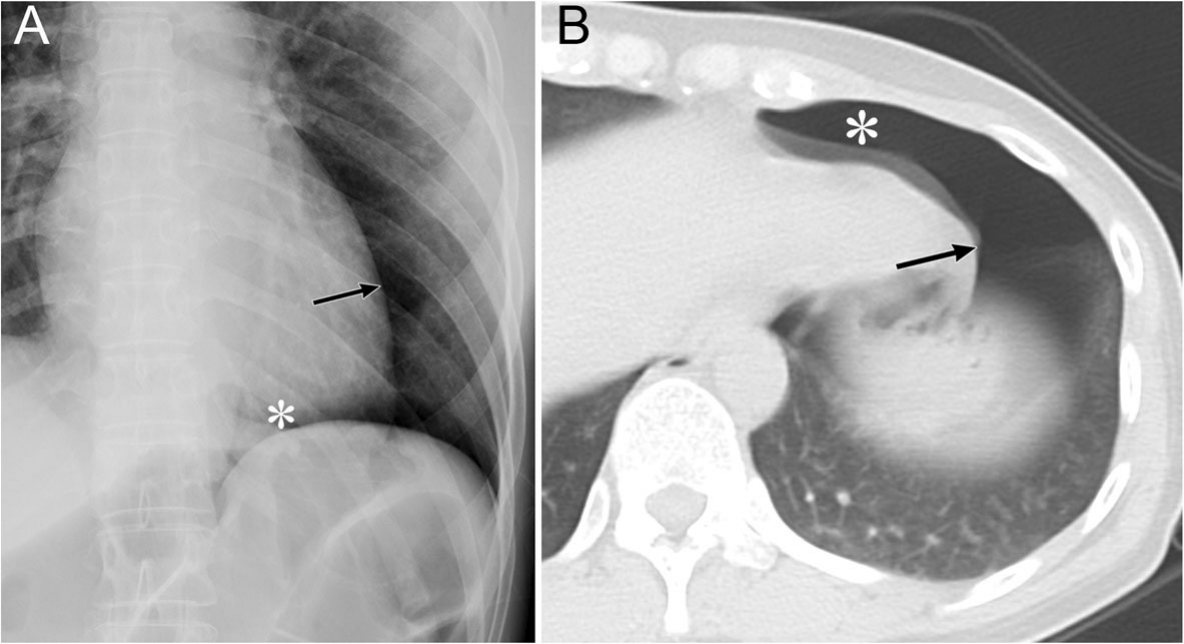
Pneumothorax in the infrahilar anteromedial space of a 39-year-old woman due to traffic accident-related trauma. a A supine radiograph shows a sharp delineation of the left heart border (arrow) and a deep anterior cardiophrenic sulcus (asterisk). b An axial CT of the lung base shows substantial air collection in the pleural cavity at the anterior cardiophrenic sulcus (asterisk) and on the left side of the heart border (arrow)
